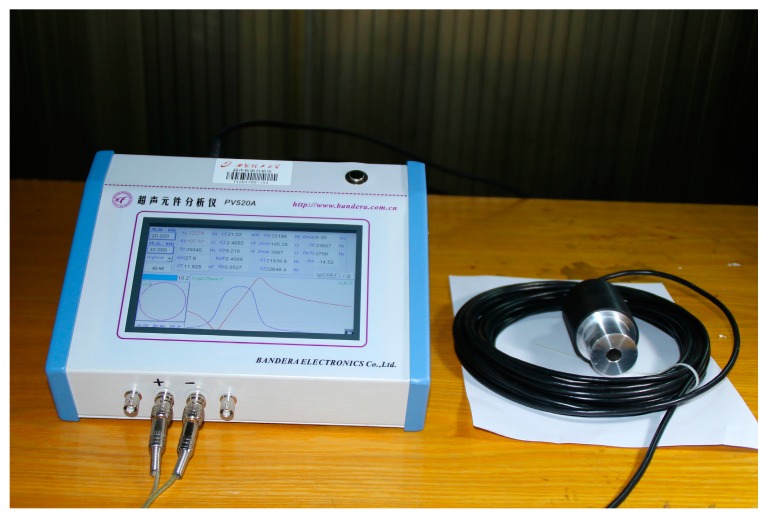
The impedance test of the sandwiched ultrasonic transducer.
